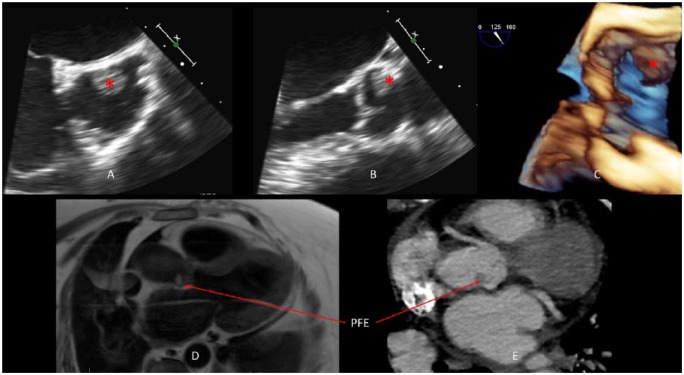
(A) Transesophageal echocardiogram (TEE) short-axis view of the aortic valve shows round echo-density mass (*), between the left and noncoronary cusps, in proximity to the left main ostium. (B) TEE long-axis view of the aortic valve as well as the ascending aorta demonstrates papillary fibroelastoma (PFE) location. (C) PFE noted on 3-dimensional TEE long-axis view of the aortic valve, aortic root, and ascending aorta. (D) T2-weighted cardiac magnetic resonance imaging confirms the density noted in the aortic root, attached to the sinotubular junction. (E) Computed tomography image of a prior scan suggestive of a mass in the aortic root.
